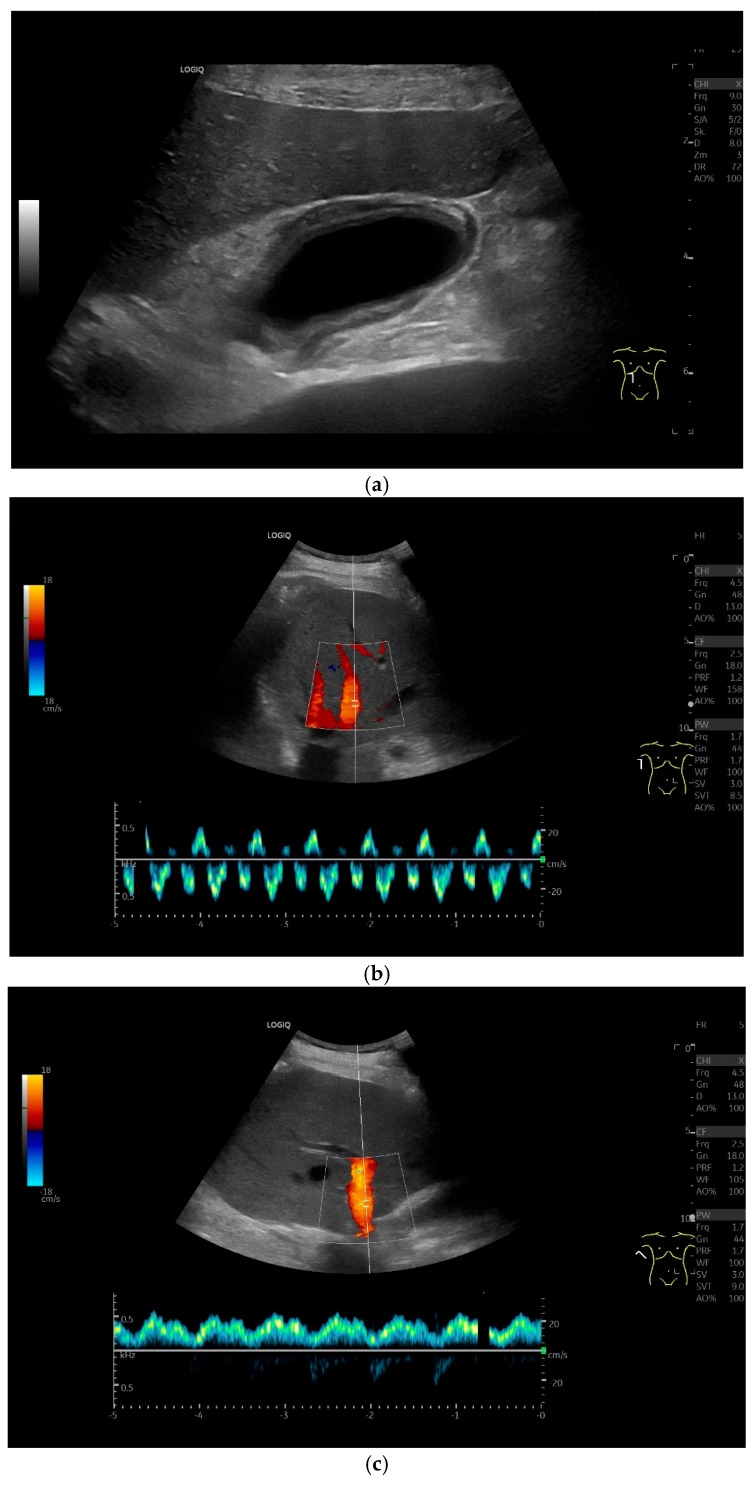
Congestive heart failure. Thickened and stratified GB wall (a), no stones, no clinical and laboratory signs of inflammation. Moderate pressure pain in the right upper abdomen due to liver congestion due to right heart failure. Pendulating flow in the hepatic veins (b) and strongly undulating flow in the portal vein (c) on Color Doppler imaging, indicating severe right heart insufficiency with trans-sinusoidal congestion. (Source: own collection, K.M.).
